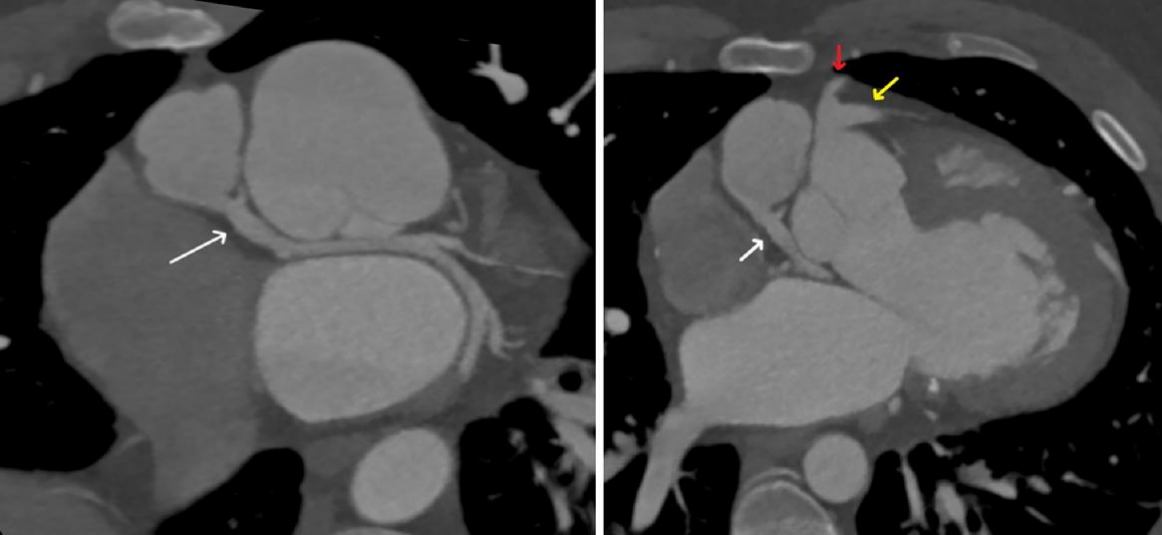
CT shows anomalous origin of the left circumflex artery arising from the pulmonary artery (left arrow) (left). CT demonstrating anomalous left circumflex artery as well as the right coronary artery ( top arrow) and left anterior descending (right arrow) arising from the right coronary cusp (right). *Source*: Mater Private Network.
